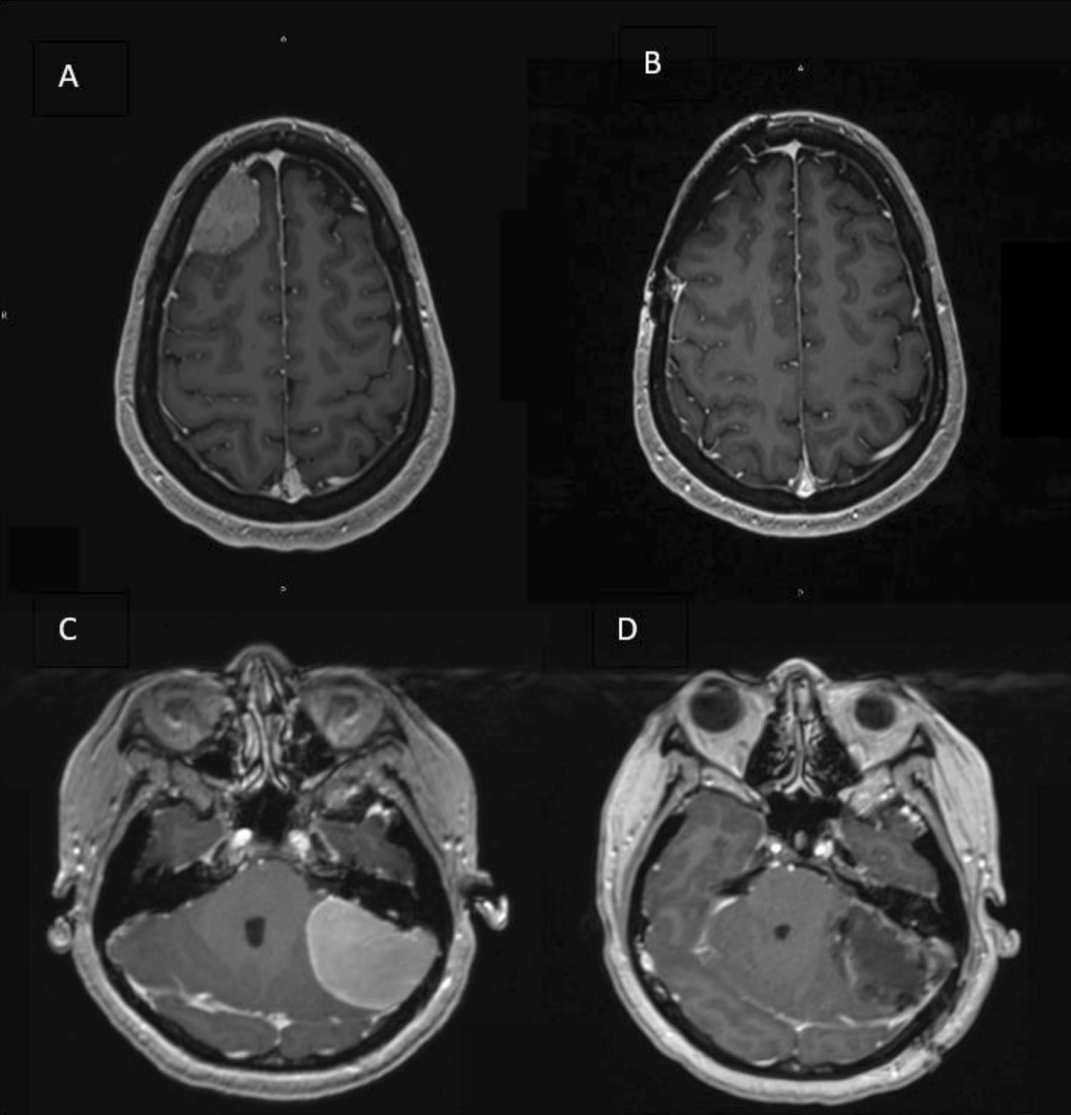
Axial views of an MRI with contrast demonstrating an *NF2* subtype meningioma of the convexity before (A) and after gross total resection (B) and a large *NF2* subtype posterior fossa meningioma before (C) and after (D) gross total resection. Despite its more benign appearance, the convexity meningioma (A, B) is a WHO grade 2 atypical tumor with NF2 mutation as well as a somatic *SUFU* mutation, genomic instability, and 65% of genome loss of heterogeneity. Conversely, the posterior fossa meningioma (C, D) appears more aggressive but is a WHO grade 1 tumor without genomic instability
